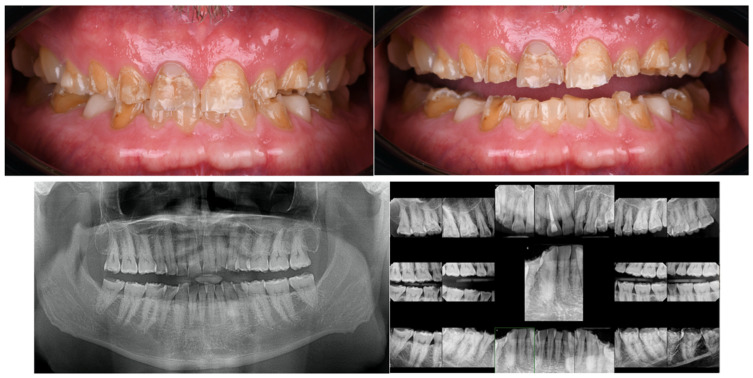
Intraoral photographs revealed a severely worn dentition with significant loss of tooth structure in the vestibular region and incisal/occlusal regions. A panoramic and full mouth series X-ray revealed severe tooth wear.
